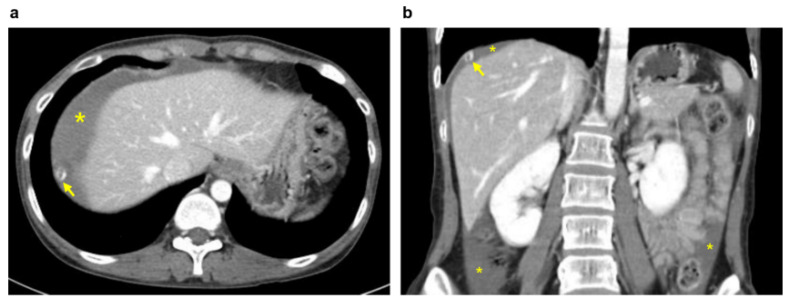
Abdominopelvic computed tomography images: A 2 cm target-like nodule with a thin, enhancing capsule (arrow); hemoperitoneum (*) in the axial (a) and coronal (b) planes.
